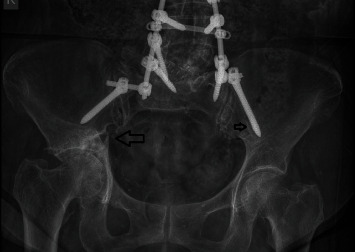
Anteroposterior pelvis roentgenogram showing the acetabular fracture (right, thick arrow) and radiolucent line around the left iliac screw (small arrow).
